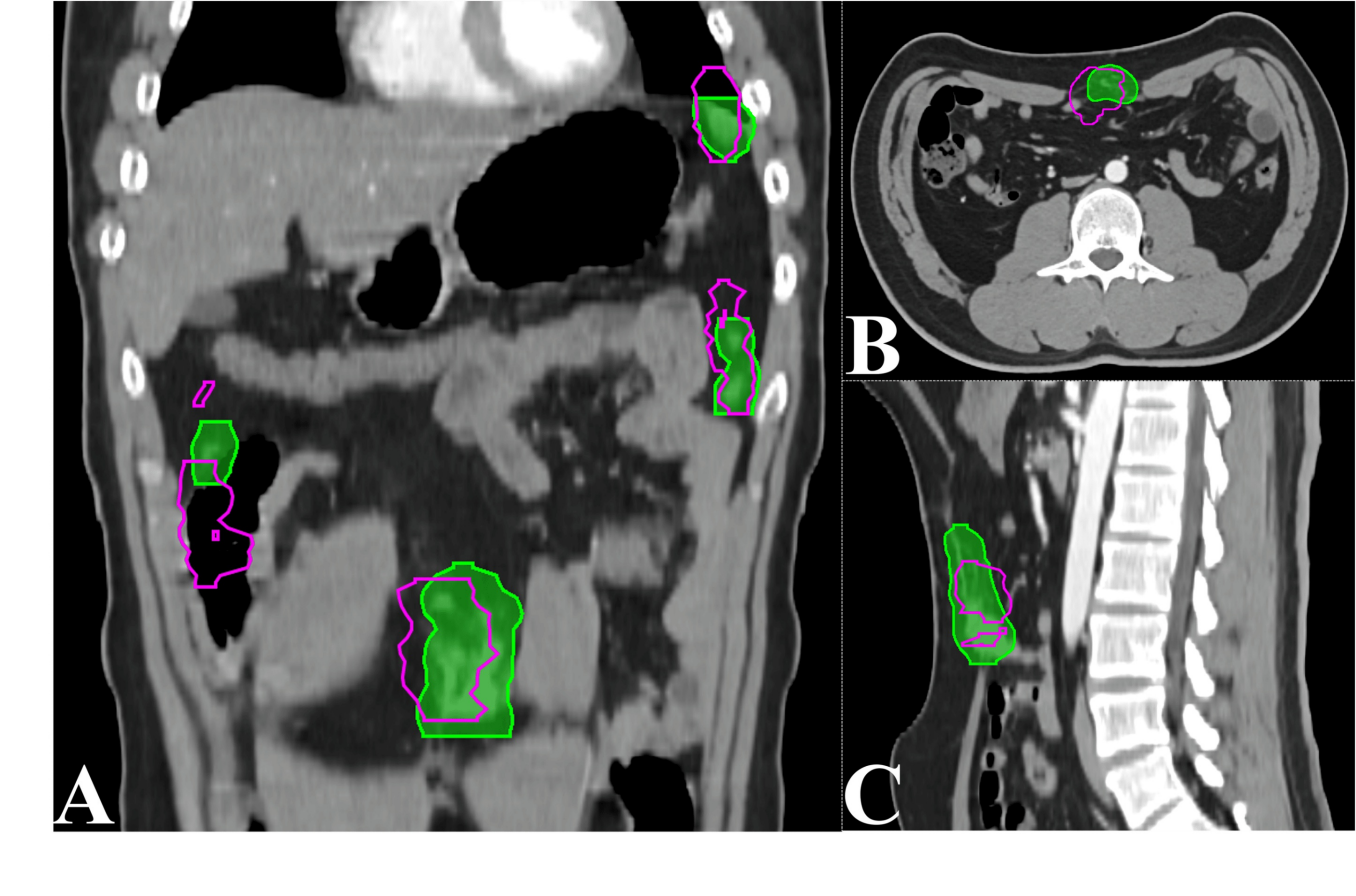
The coverage of the planning CT's PTV for the GTV over 25 fractions A, B, C are planning CT images in coronal, transverse, and sagittal views. The purple lines represent re-delineated GTV delineations from 25 fractions of ART, and the green region represents the PTV from the baseline treatment plan. *Abbreviations:* CT: Computed tomography; PTV: Planning target volume; GTV: Gross tumor volume; ART: Adaptive radiotherapy.
